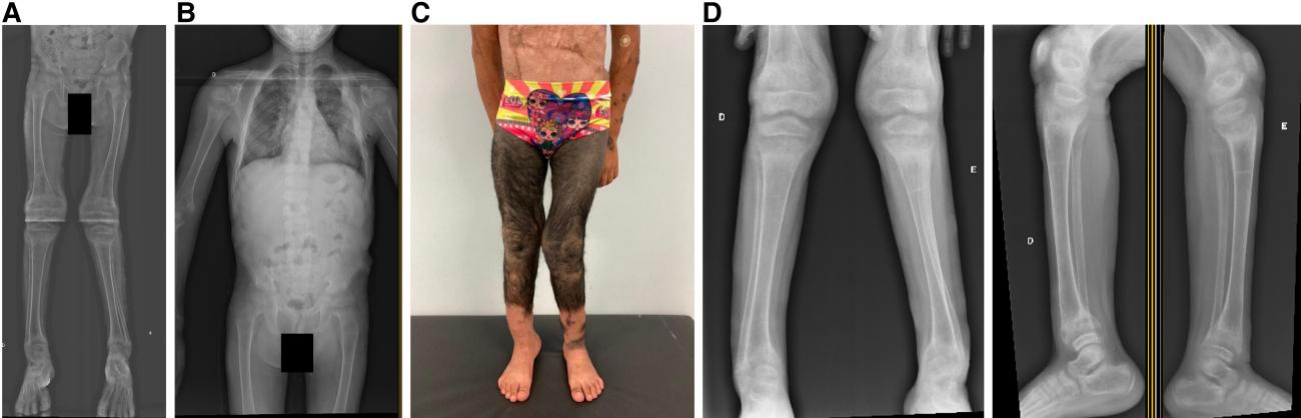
A, X-ray of the lower limbs, and B, panoramic x-ray of the spine. C, Patient presents with giant hairy nevus distributed on both legs and surgery scars in the abdomen region. D, Valgus knee and metaphyseal enlargement are shown in the radiographic images.
